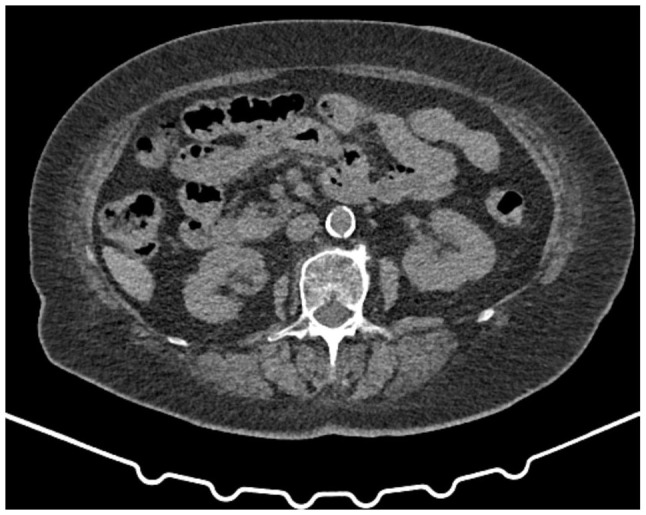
Lower sections of the thoracic computed tomography scan revealed no abnormalities in the renal parenchyma and collecting system.
